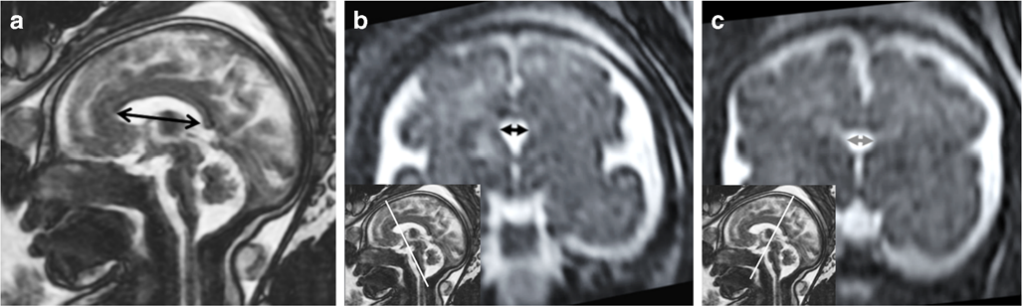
Reconstructed images from T2-weighted volume imaging of a foetal brain at 31 gw. a The midline sagittal image showing how the cranio-caudal length of the CSPV was measured (double-headed arrow). b A coronal image showing how the coronal width of the cavum septi pellucidi was measured (double-headed arrow on b) with an inset to show the coronal plane used. c The equivalent images for measuring the width of the cavum vergae
